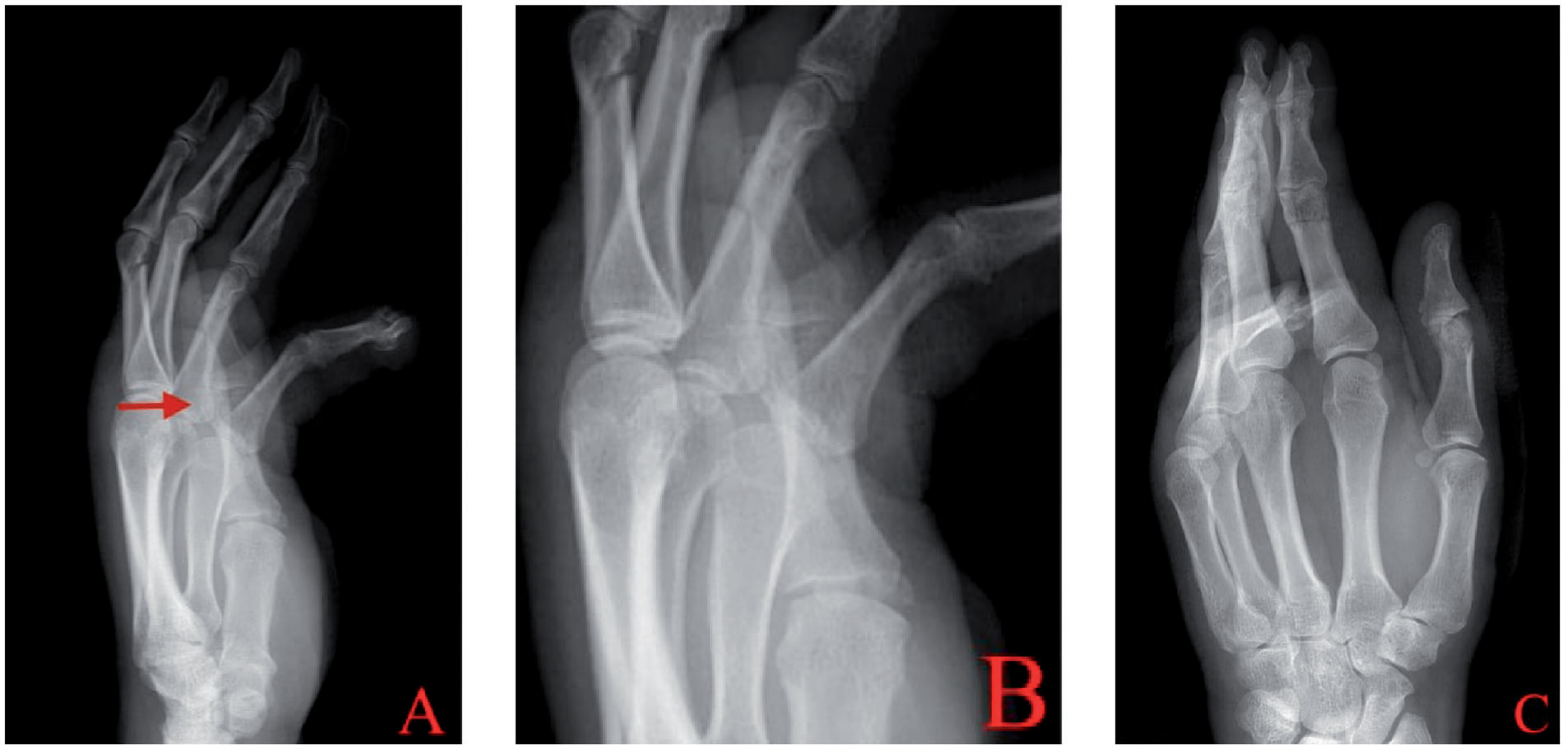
Injury radiographs anteroposterior and lateral of hand (A, C) with focus on thumb (B) demonstrate a condylar fracture of proximal phalangeal head (arrow).
